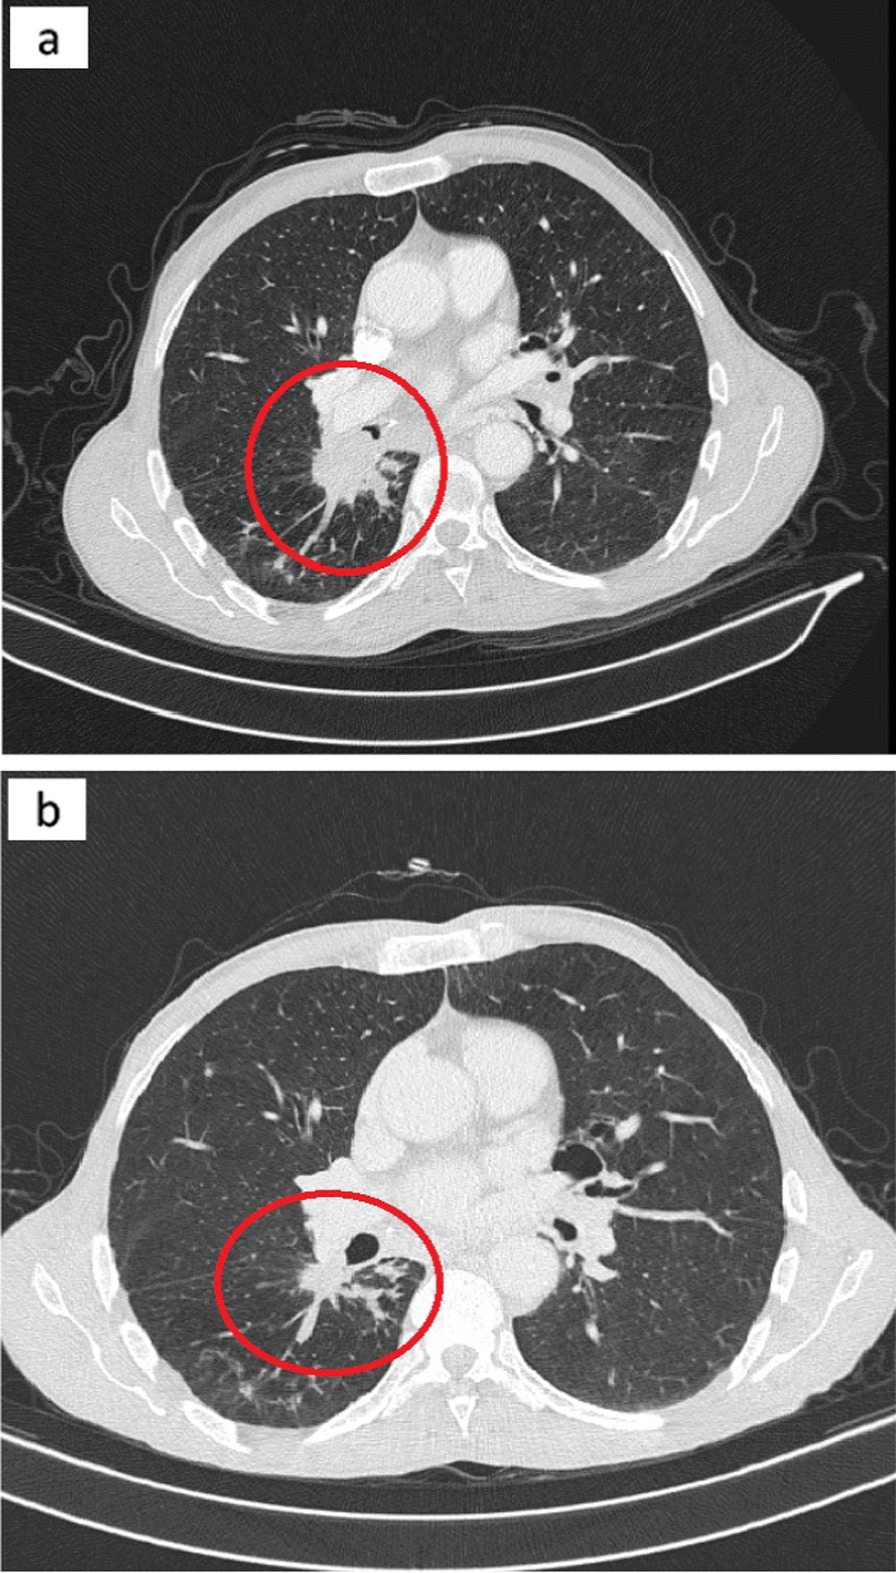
CT scan results showing the primary tumor in the right lower lobe of the lung before treatment (a), and the dramatic response 3 months post-afatinib treatment (b). The red circle represents the primary tumor before and after treatment
